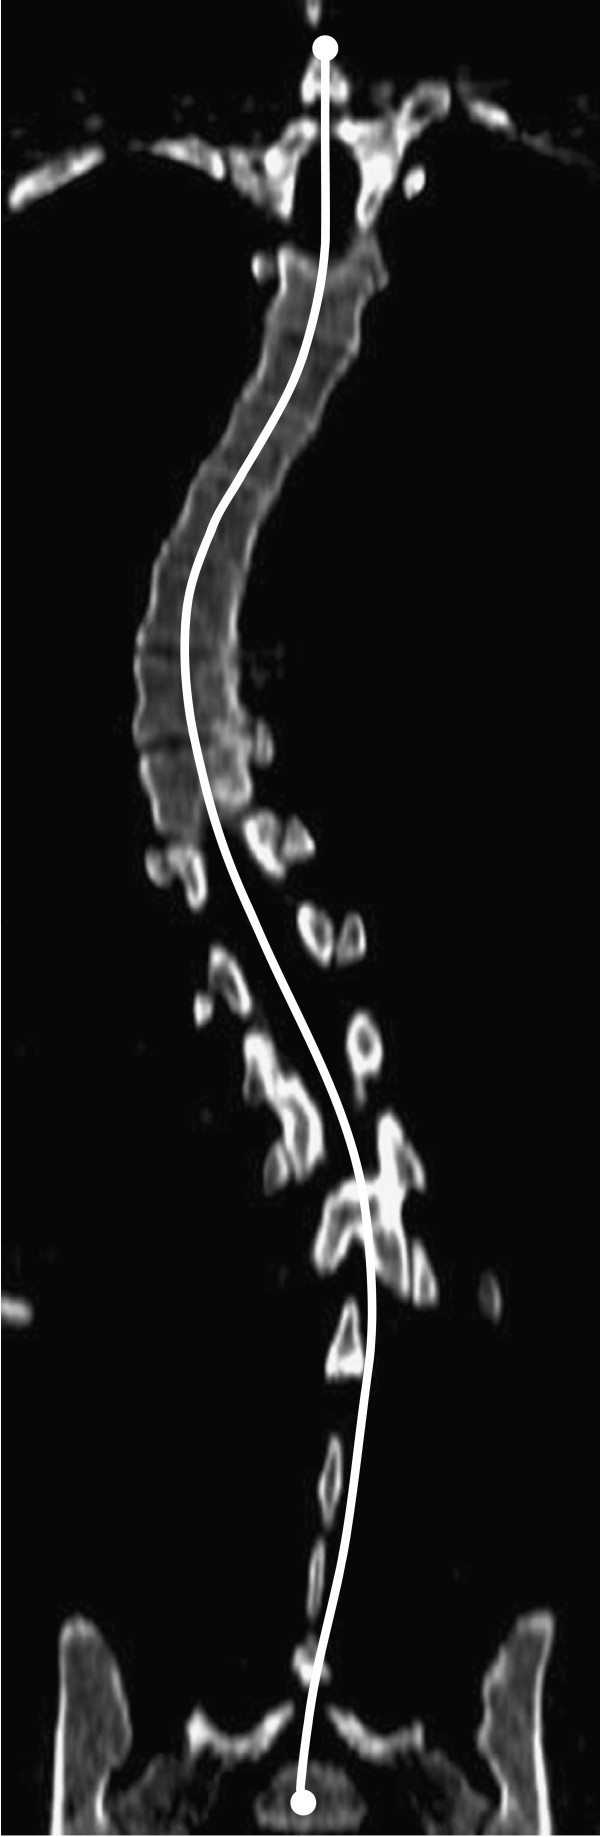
Method of reformatting CT scan dataset to obtain sagittal section by tracing the path of the mid-sagittal plane to account for the scoliotic curvature in the coronal plane.
